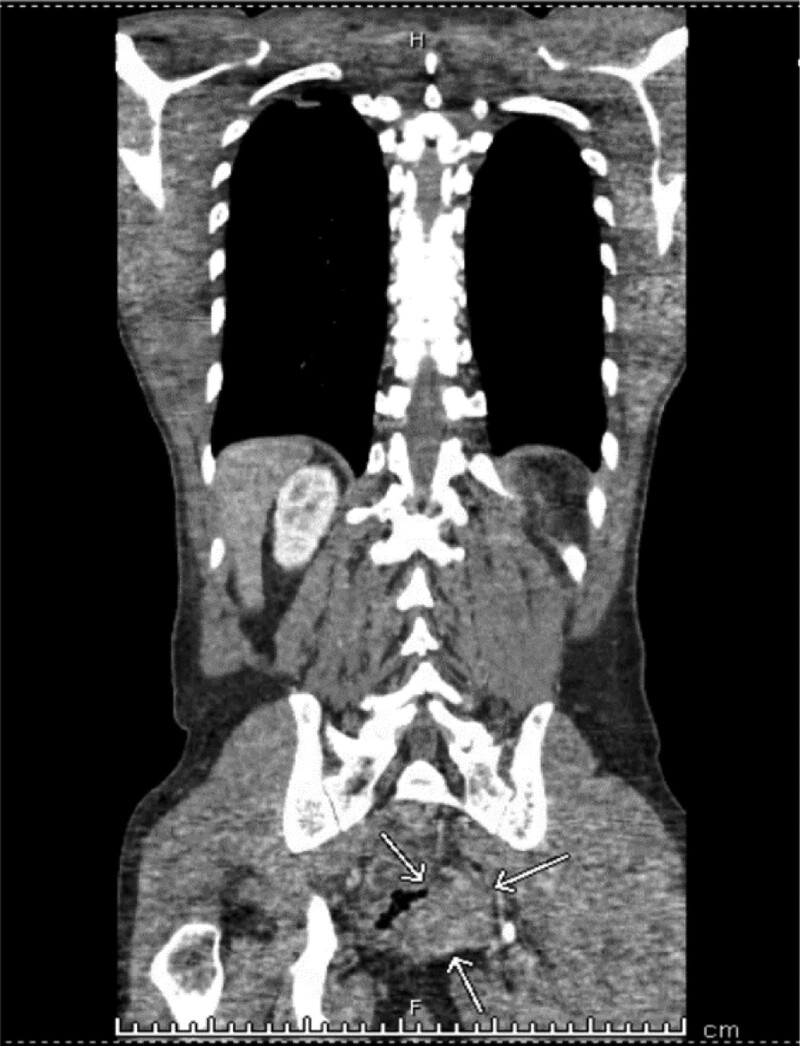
CT abdomen and pelvis with IV contrast showing 4.1 × 5.6 cm enhancing soft tissue mass in the left posterior wall of the rectum (thin white arrows).
